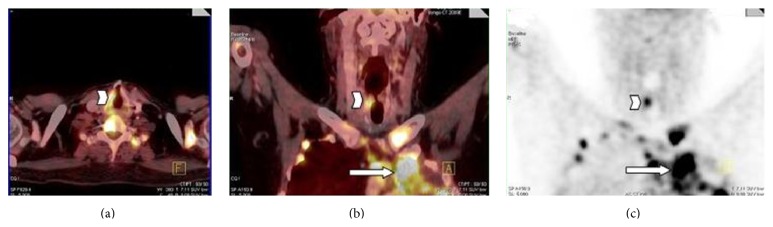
Second PET/CT: (a) transverse, (b) coronal PET/CT fusion, and (c) coronal PET images show intense FDG uptake in the left lung tumor (arrow) and in the right vocal cord (arrowhead).
